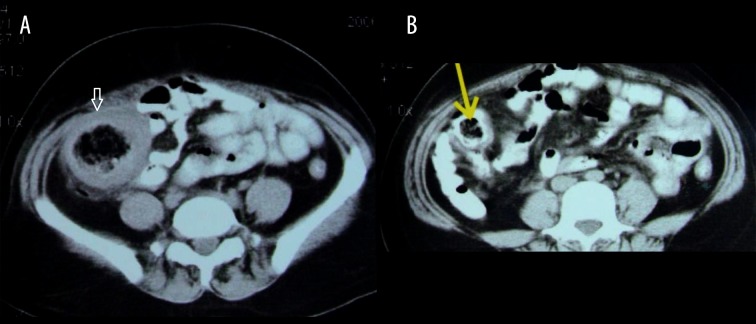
(A) Contrast-enhanced CT scan shows a thick-walled lesion in the right iliac fossa with central, mottled gas attenuation. (B) More caudal section reveals that the mass have migrated into the caecum.
